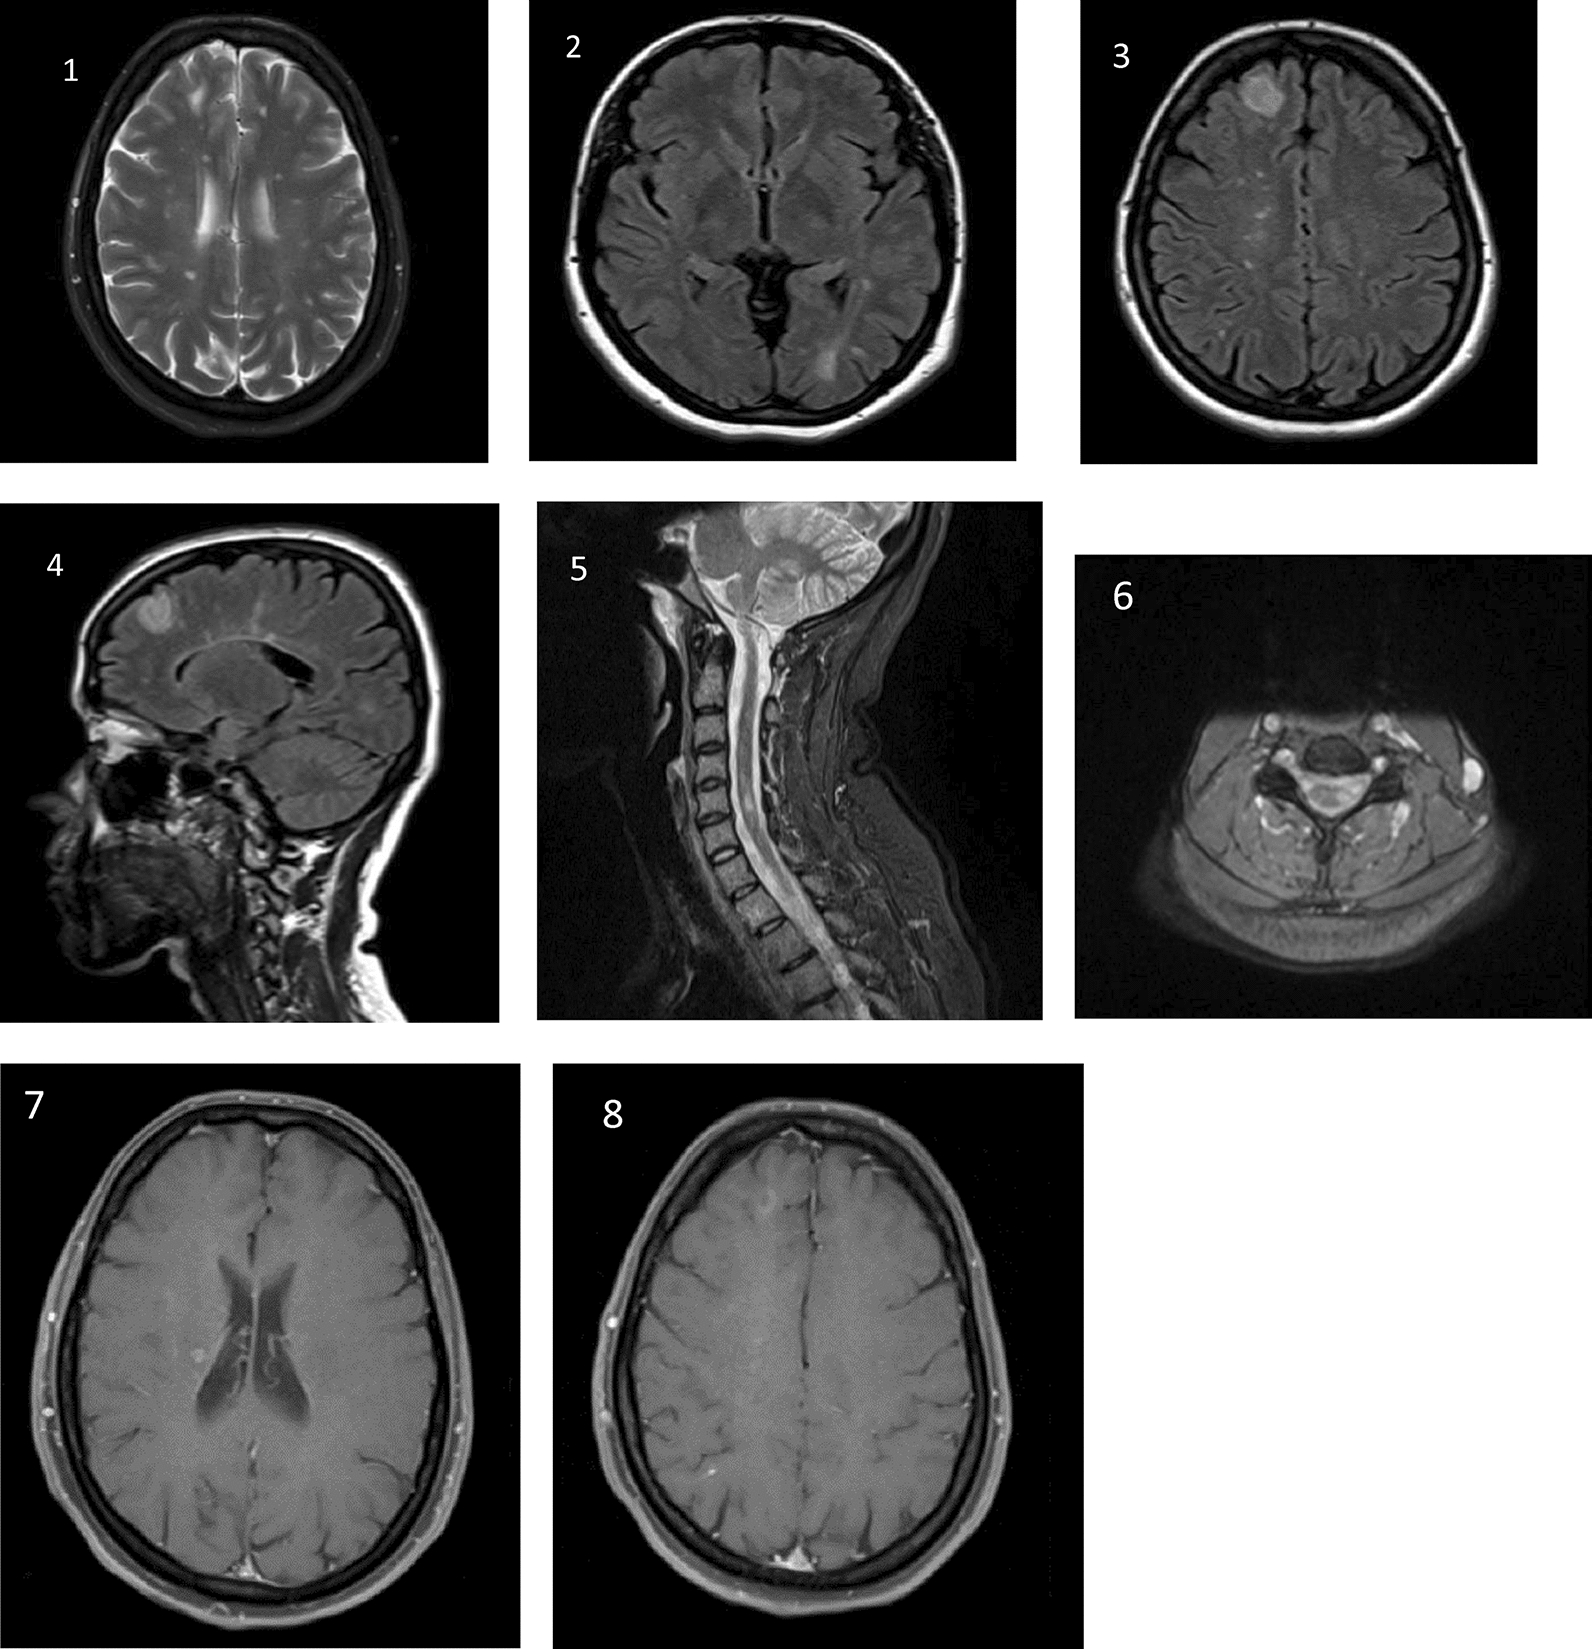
MRI images: axial (1[T2]) and (3[flair]) images showing juxtacortical plaques, axial (2[flair]) showing periventricular plaques, sagittal (4[sagittal]) image showing tumefactive juxtacortical plaques, and (5[stir]) showing cervical plaques. Axial (6) shows spinal plaques, and (7) and (8) show gadolinium-enhanced lesions in brain
